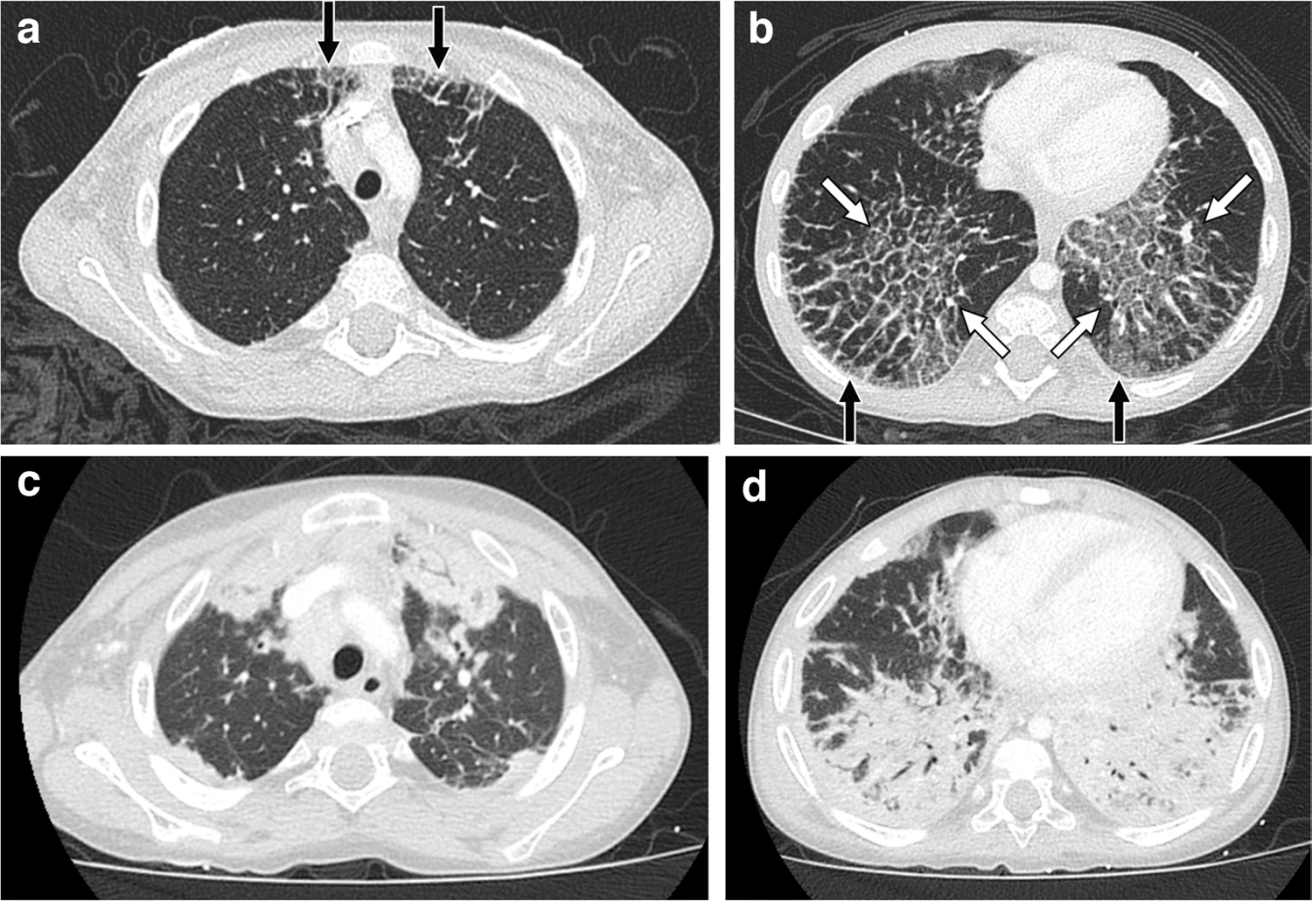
2-year-old male with systemic juvenile idiopathic arthritis. a, b Axial CT images with intravenous contrast showing ground-glass opacity, interlobular septal thickening, and intralobular lines in a crazy paving pattern in a subpleural distribution in the anterior upper lobes and peripheral lower lobes (black arrows), and in a peribronchovascular distribution in the lower lobes (white arrows). c, d Follow-up axial CT images with intravenous contrast at 5 years of age showing extensive consolidation at sites of prior ground-glass opacity and interlobular septal thickening
